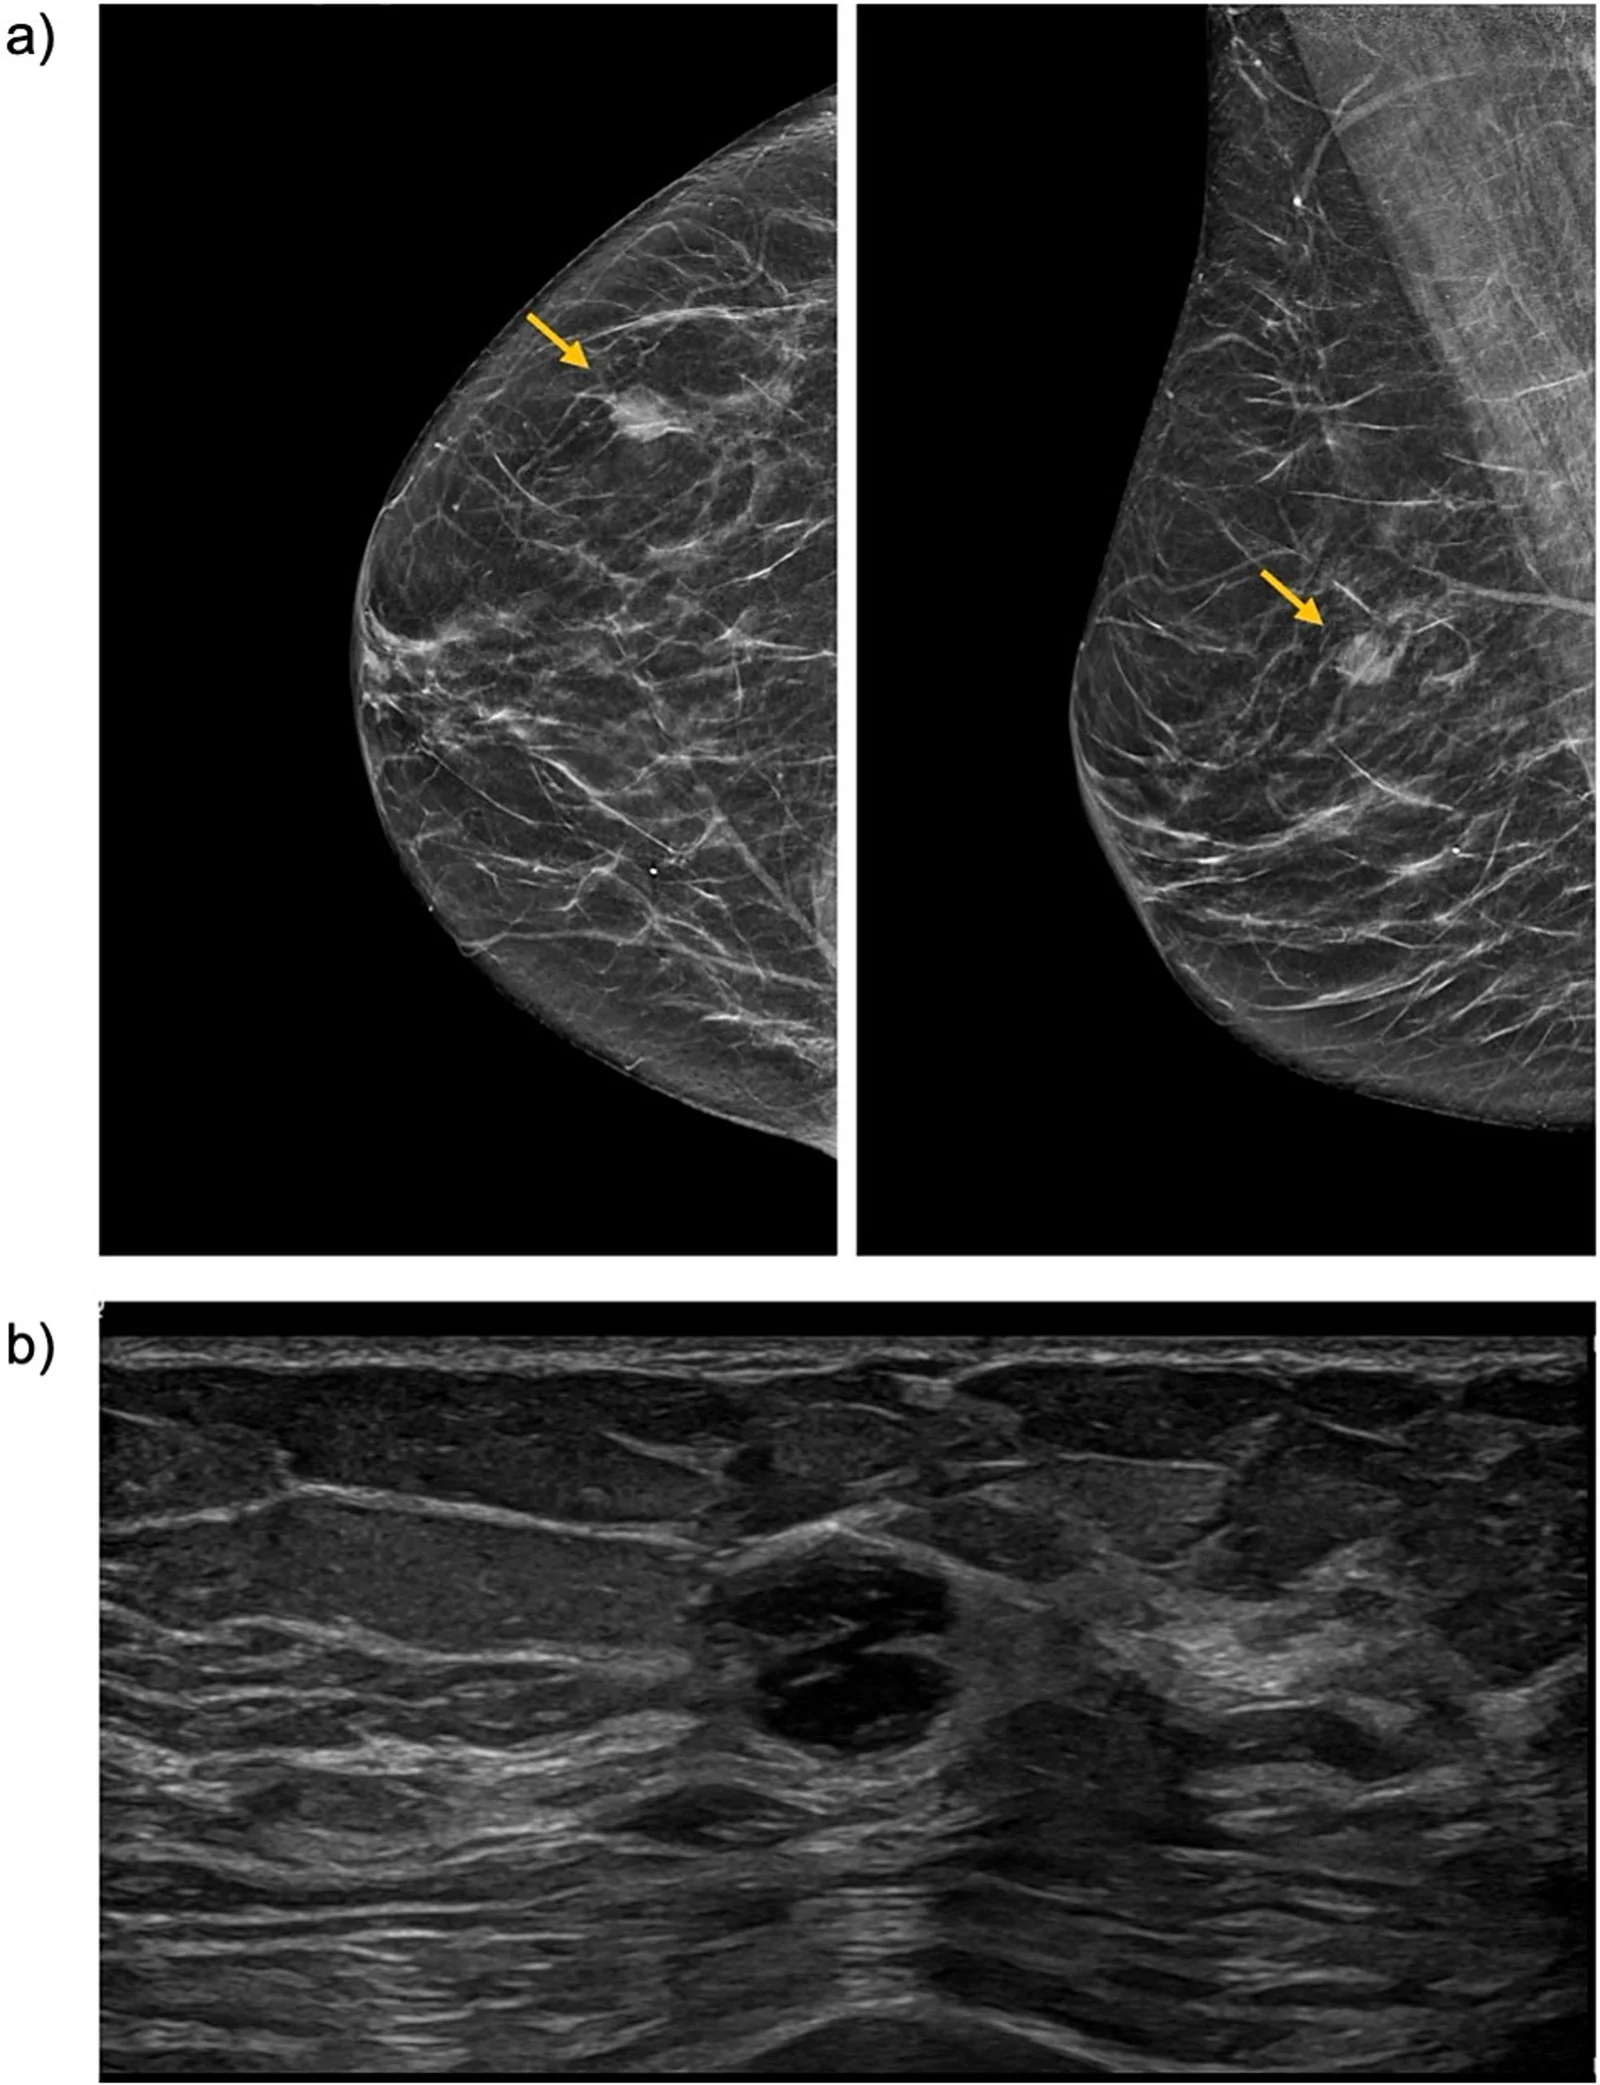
(a) Mammographic images showing 1 cm oval mass in the outer right breast. (b) Ultrasound of hypoechoic oval cystic and solid mass with posterior acoustic enhancement measuring 1.0 × 0.7 × 0.8 cm.
